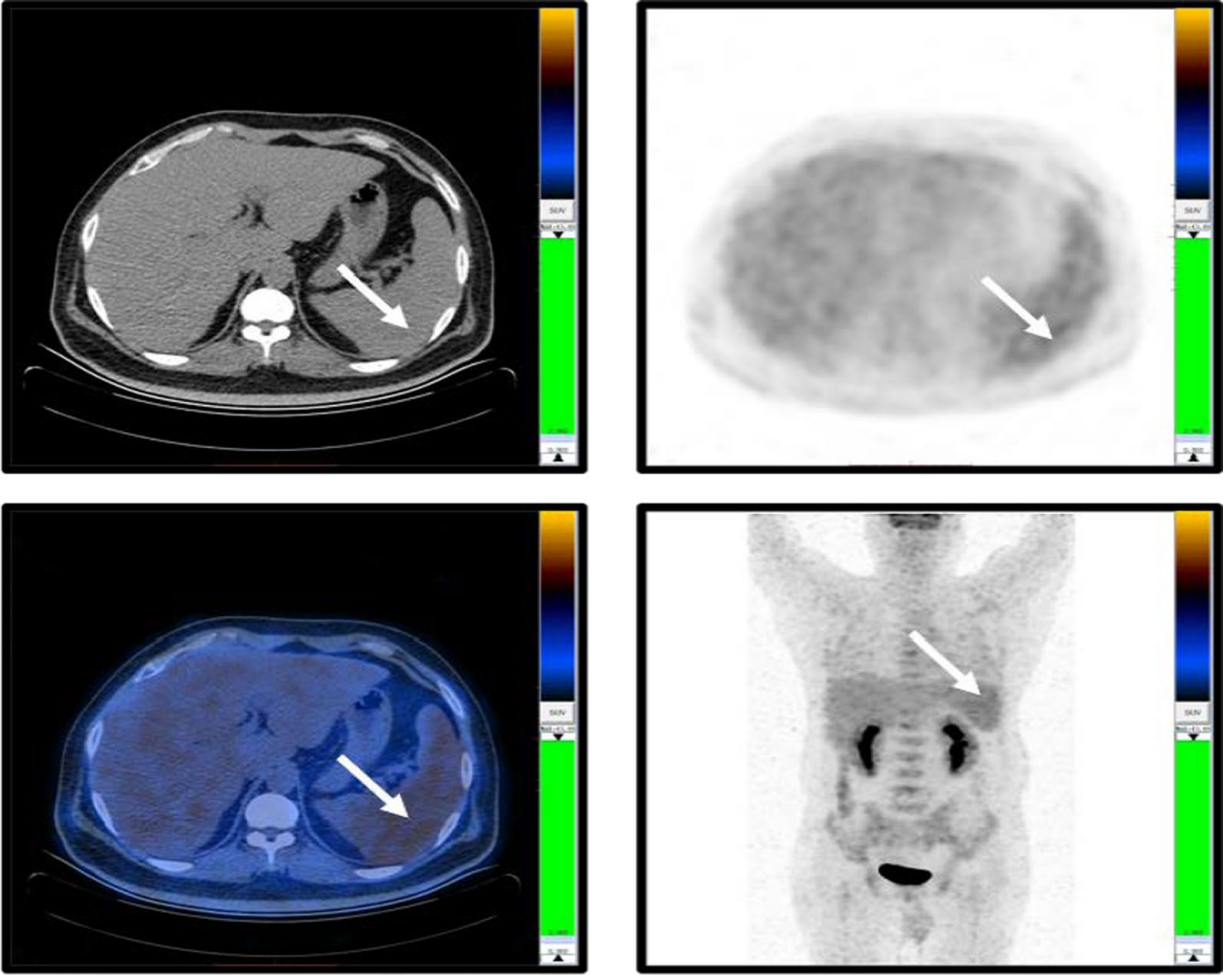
PET-CT images show splenomegaly (about 7 rib units), and slightly increased glucose metabolism (SUV_max_ 4.1)
